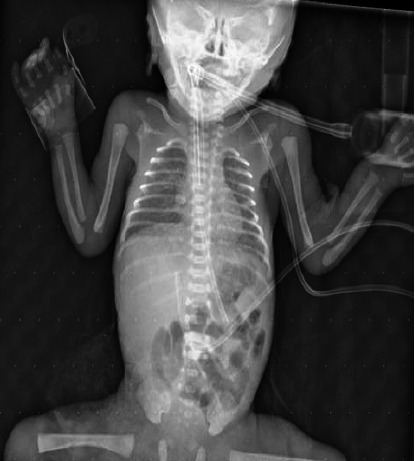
The initial chest x-ray demonstrated diffuse reticulogranular opacities with air bronchograms, consistent with neonatal respiratory distress syndrome (RDS). There was no evidence of pneumothorax or congenital lung anomalies.
